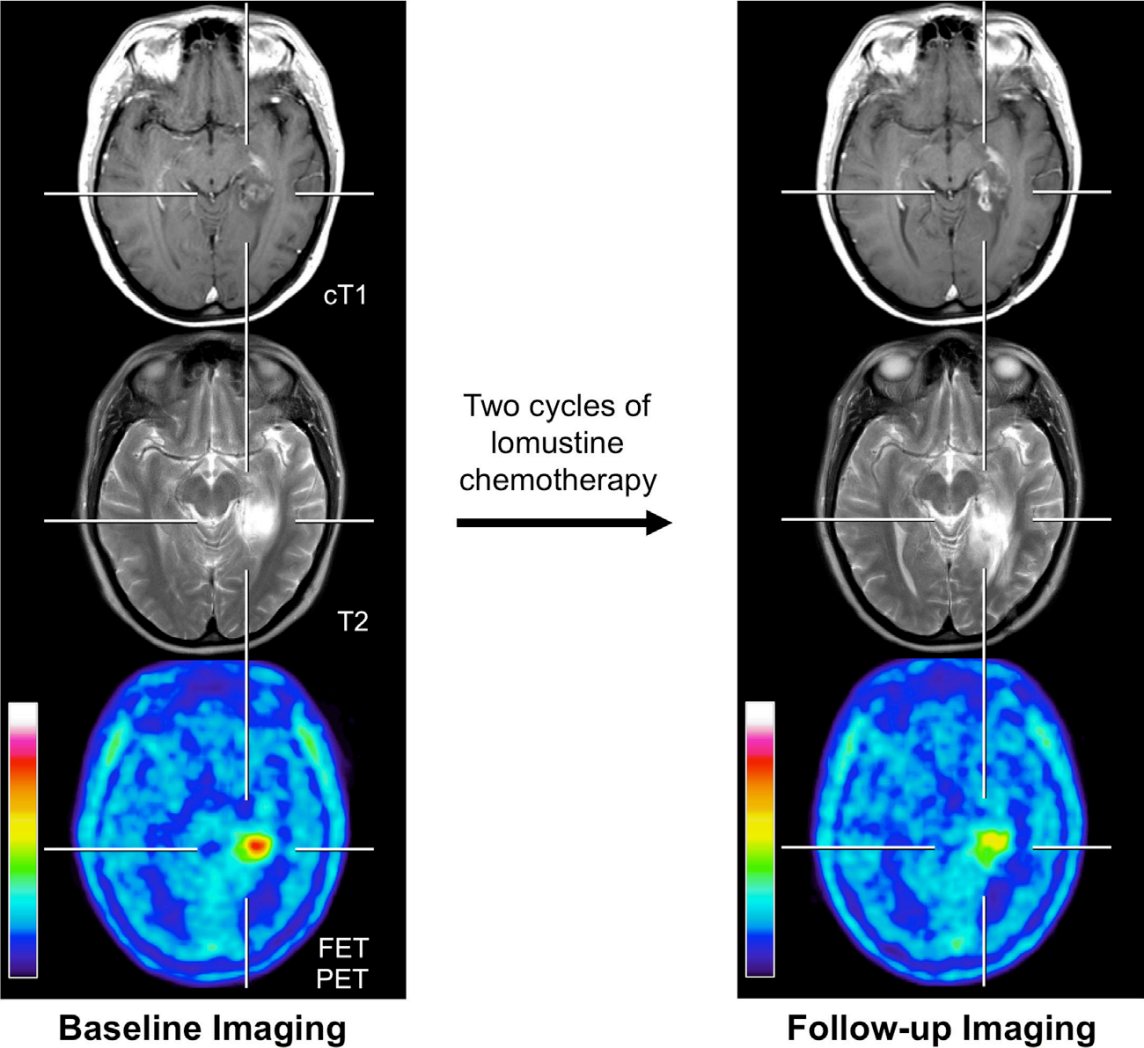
Example of a 31-year-old patient with an anaplastic astrocytoma in the left parahippocampal region with posttherapeutic effects. Previous treatment consisted brachytherapy and external fractionated radiotherapy with concomitant and adjuvant temozolomide. Brain imaging with standard MRI and FET PET at recurrence/before initiation of chemotherapy (left column) and 4 months later after two cycles of lomustine chemotherapy (right column). Enlargement of contrast enhancement and the T2 signal is suggesting tumor progression, whereas FET PET indicates a reduction of metabolic activity.
